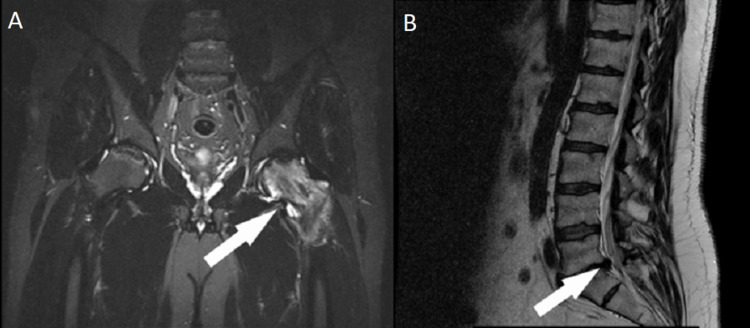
MRI: (A) Coronal plane, MRI showing avascular osteonecrosis of the left femoral head, and (B) sagittal plane, MRI showing disc herniation at L5-S1
